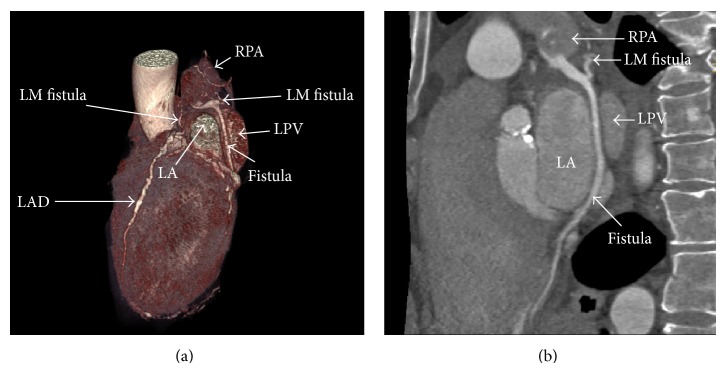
(a) Volume rendering CTA showed LM fistula joining the fistulous vessel of the other coronary arteries draining into the RPA. (b) Curved MPR showed the insertion point of the fistula at RPA. LAD: left anterior descending artery. LPV: left pulmonary vein. LA: left atrium.
